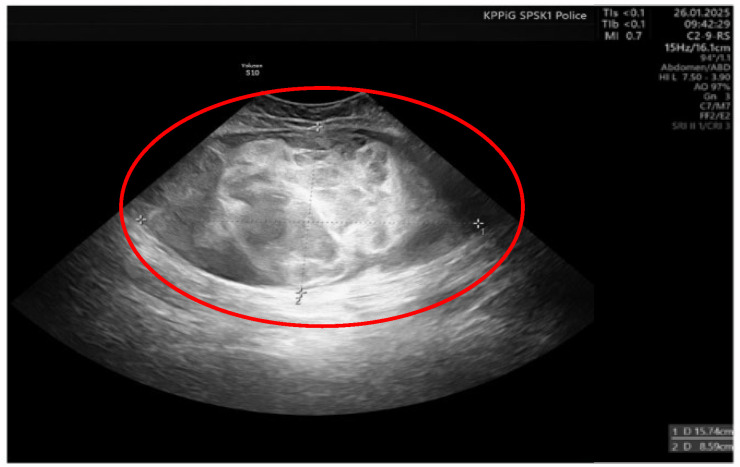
Ultrasound image of right thigh tumor (GE Voluson S10 Expert; BT16, 2019; 3/4D convex probe RAB6-RS) Red circle- tumor.
